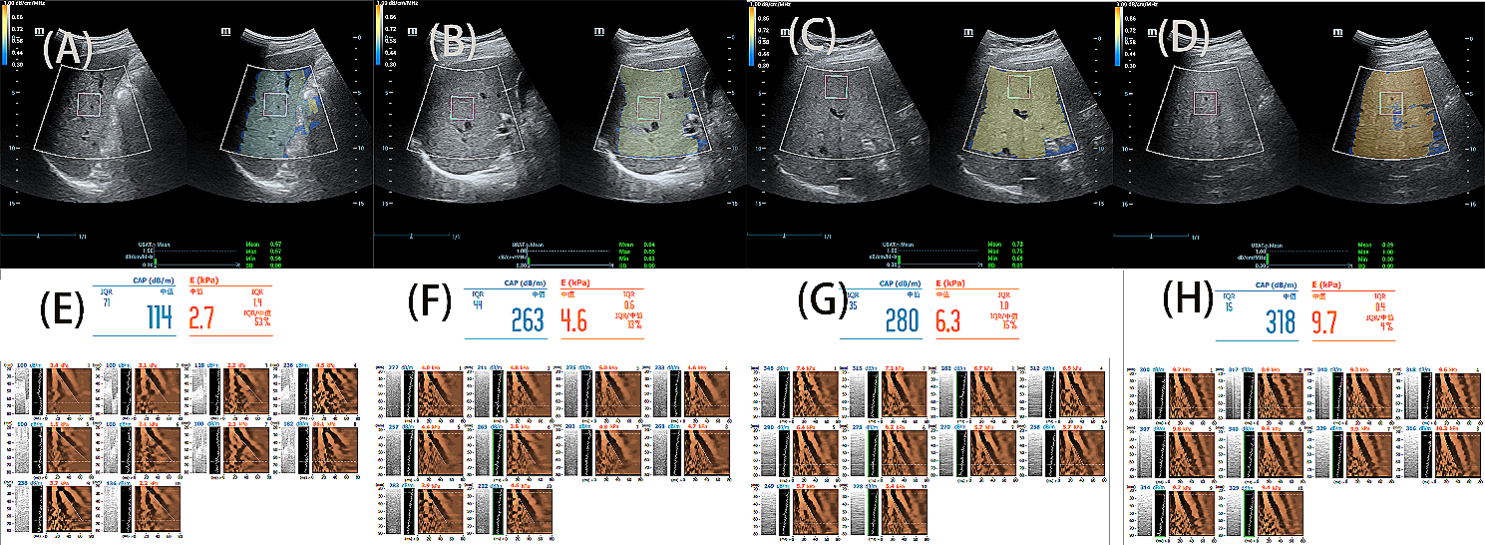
Samples of four subjects. From A to D, representing USAT measurements for no, mild, moderate, and severe hepatic steatosis; from E to H, representing CAP measurements for no, mild, moderate, and severe hepatic steatosis. CAP, controlled attenuation parameter; USAT,ultrasound attenuation analysis
